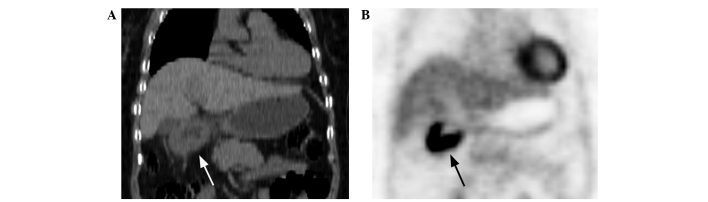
A 79-year-old female with gastric mucosa-associated lymphoid tissue lymphoma. (A) In the coronal computed tomography image, a segmental thickened gastric wall can be observed in the antrum of the stomach. (B) In the coronal positron emission tomography image, segmental increased ^18^F-fluorodeoxyglucose accumulation (maximal standard uptake value, 11.6) can be confirmed in the corresponding gastric region.
